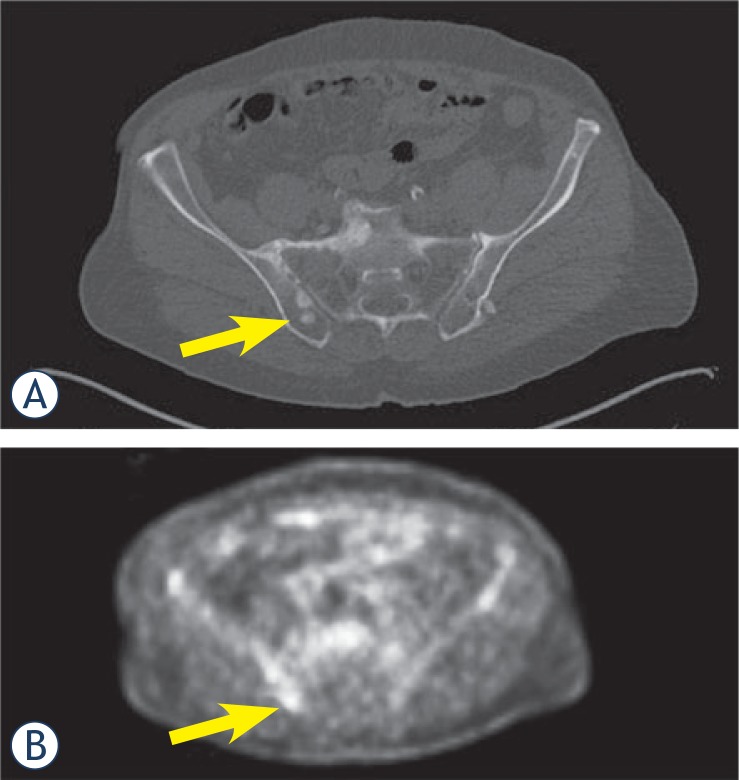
A. CT scan shows multiple osteoblastic metastases in the right iliac bone (arrow); B. PET-scan confirms these lesions to be metabolically active metastatic lesions (arrow).
